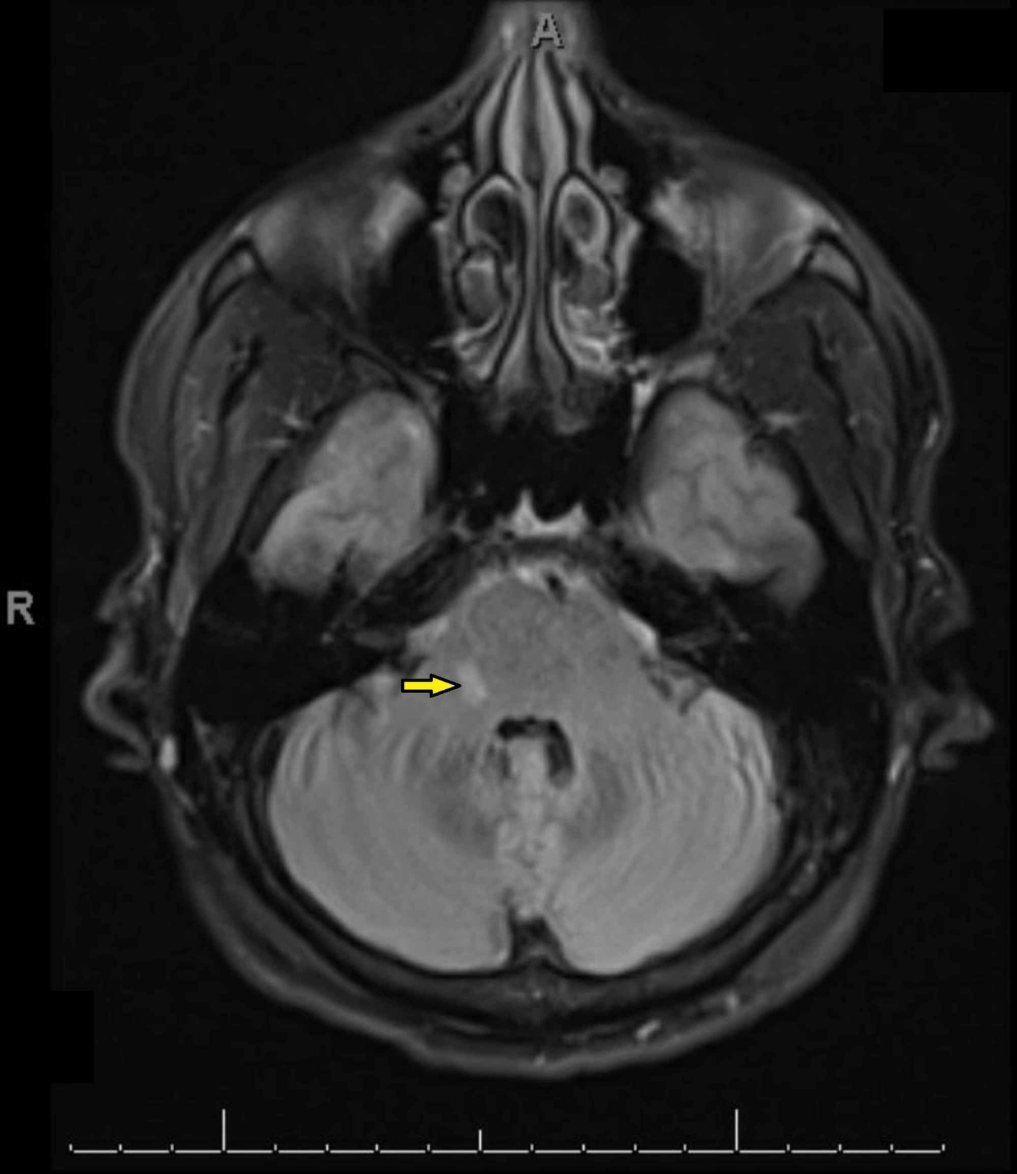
FLAIR/T2 weighted hyperintense signal foci in the right brachium pontis on MRI.
